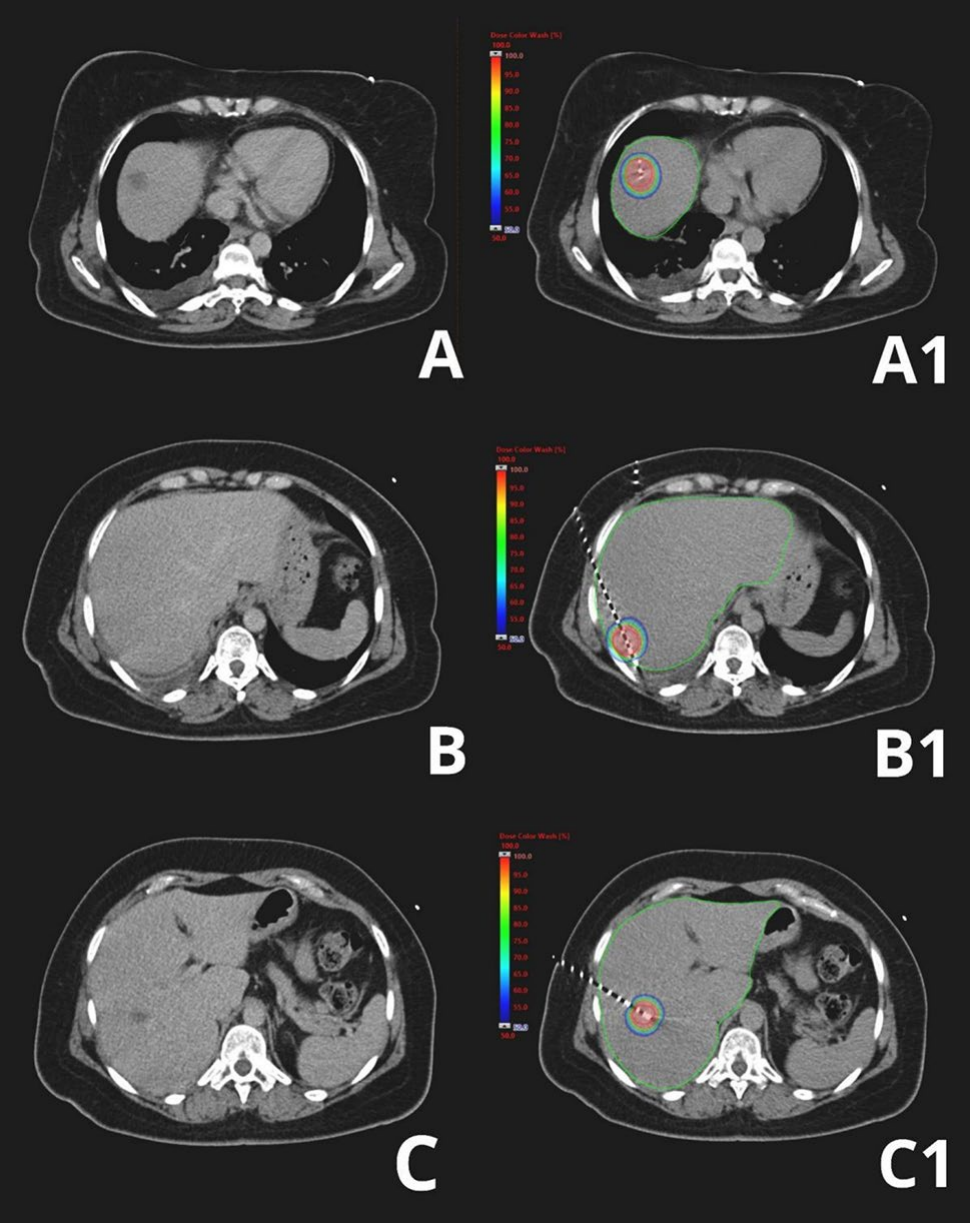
Example of one of the selected patients treated with computer tomography (CT)-guided high-dose rate brachytherapy (BRT): diagnostic CT images of three different metastases before procedure (A, B, C), and dose distribution profiles (A1, B1, C1)
